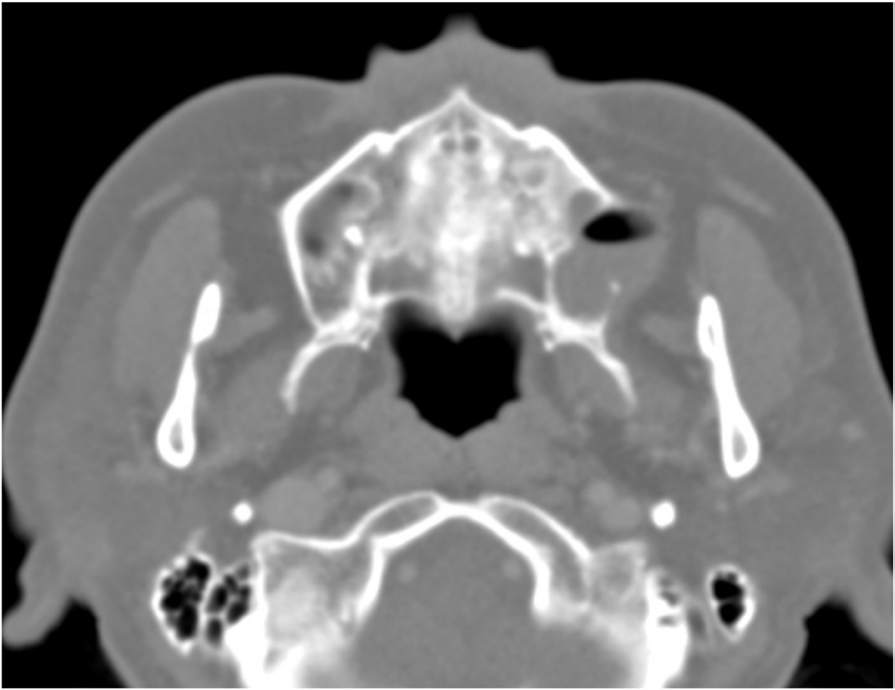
CT imaging showing a large cystic lesion invading the left maxillary sinus, with buccal cortical expansion and perforation
